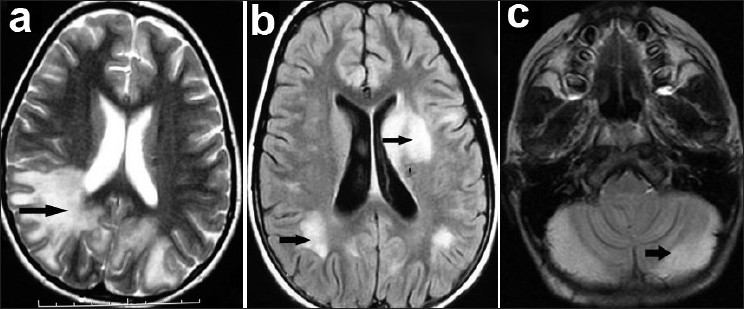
MRI of brain in CIDD. (a) T2-W axial image of ADEM patient showing hyperintense white matter signal changes in right parieto-occipital and left occipital lobes. (b) FLAIR axial cut of same patient showing hyperintense signals in left caudate nucleus, and subcortical white matter. (c) Axial FLAIR image of cerebellitis patient showing hyperintensity and swelling of both cerebellar hemispheres compressing the brainstem and occluding fourth ventricular outlet.
